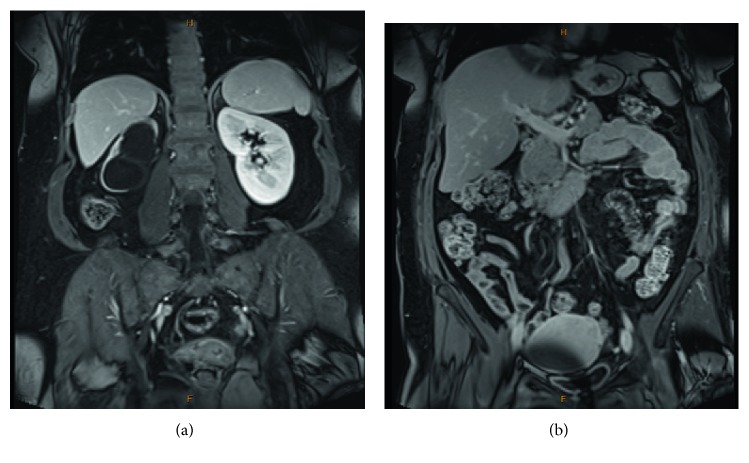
MRI confirmed significant right hydronephrosis with marked thinning of the renal parenchyma (a), and right hydroureter (b).
